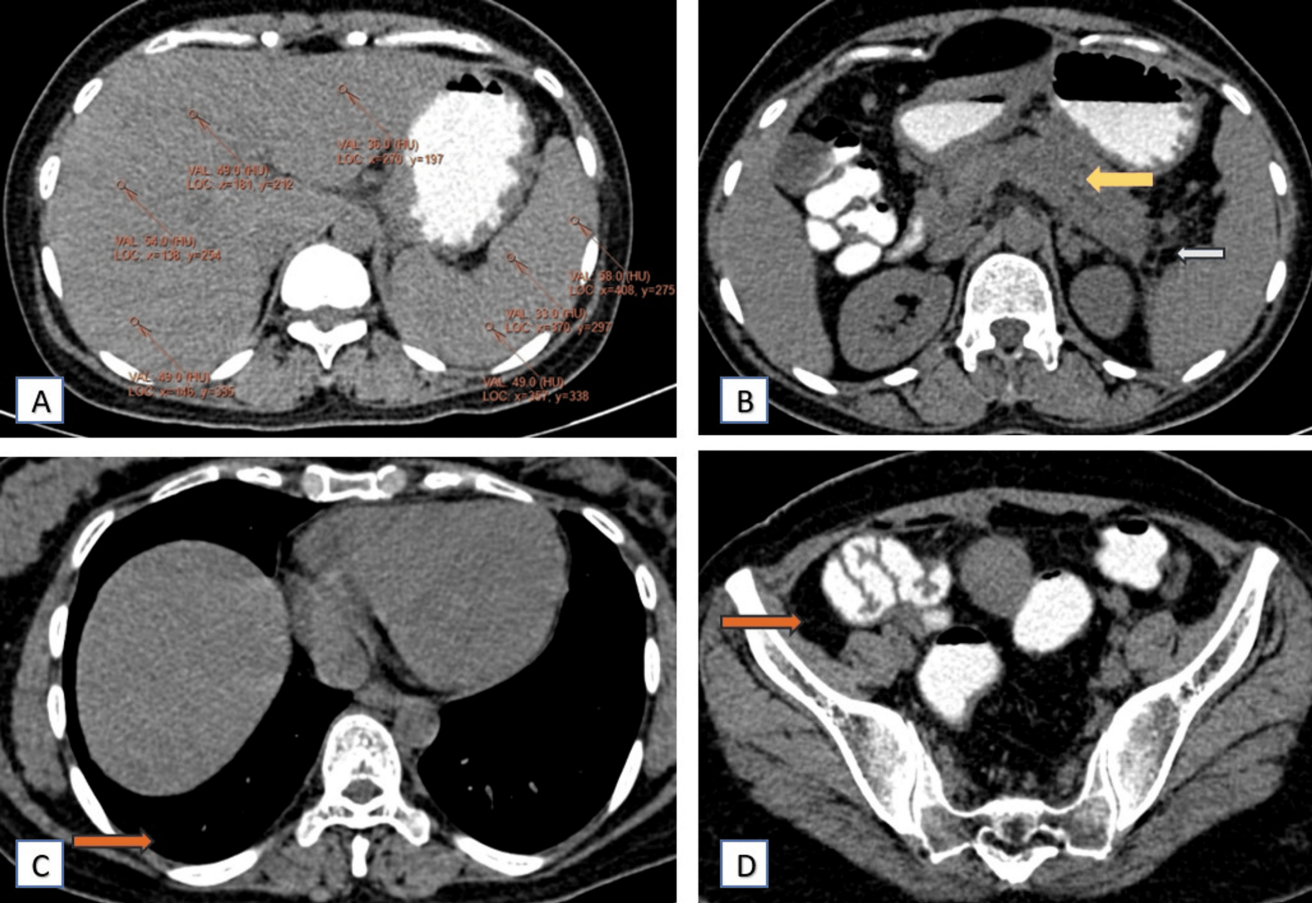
Axial plain with oral contrast CT scan images depicting regression of fatty liver and significant regression of necrotizing pancreatitis, taken after two months of conservative treatment for acute necrotizing pancreatitis Axial plain with oral contrast CT scan images depict normal attenuation of the liver compared to the spleen (A), the pancreas appears normal (yellow arrow) with complete regression of peripancreatic fat stranding and normal left lateral conal fascia (white arrow) (B), complete regression of bilateral pleural effusion (orange arrow) (C), and no free fluid in the pelvis region (orange arrow) (D). CT: computed tomography
